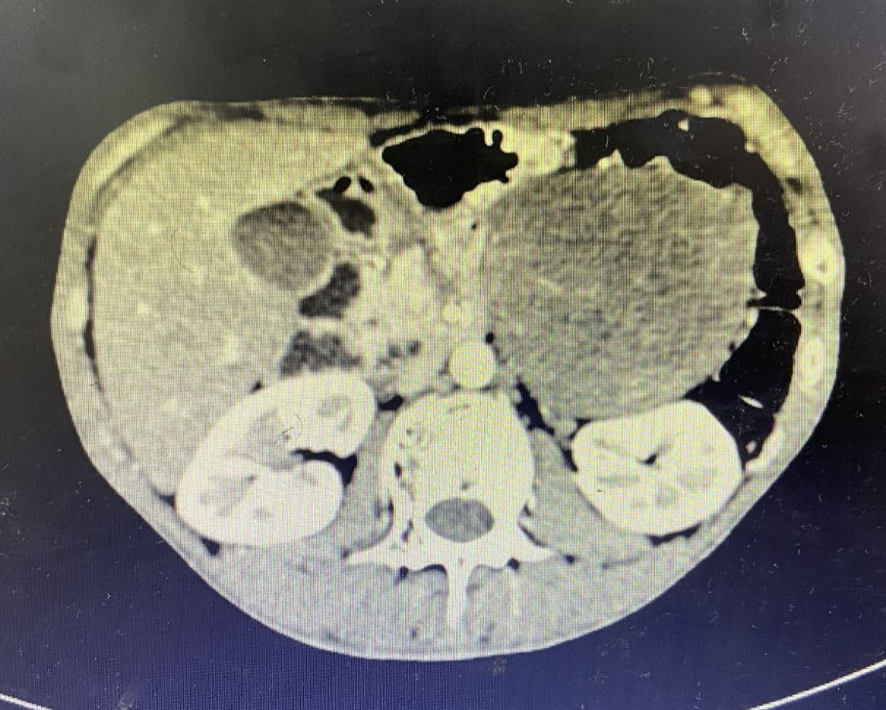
MRI imaging characteristics. Right iliac bone destruction with a 76×58×67 mm mass.
